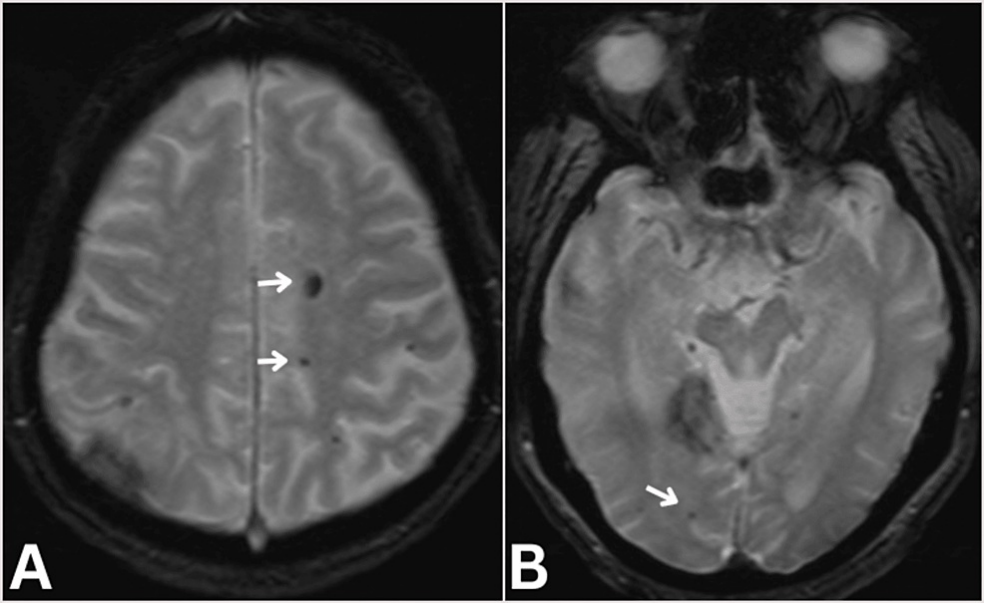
Brain GRE MRI Brain Gradient-echo GRE MRI (axial) showing diffuse microbleeds (arrows).
